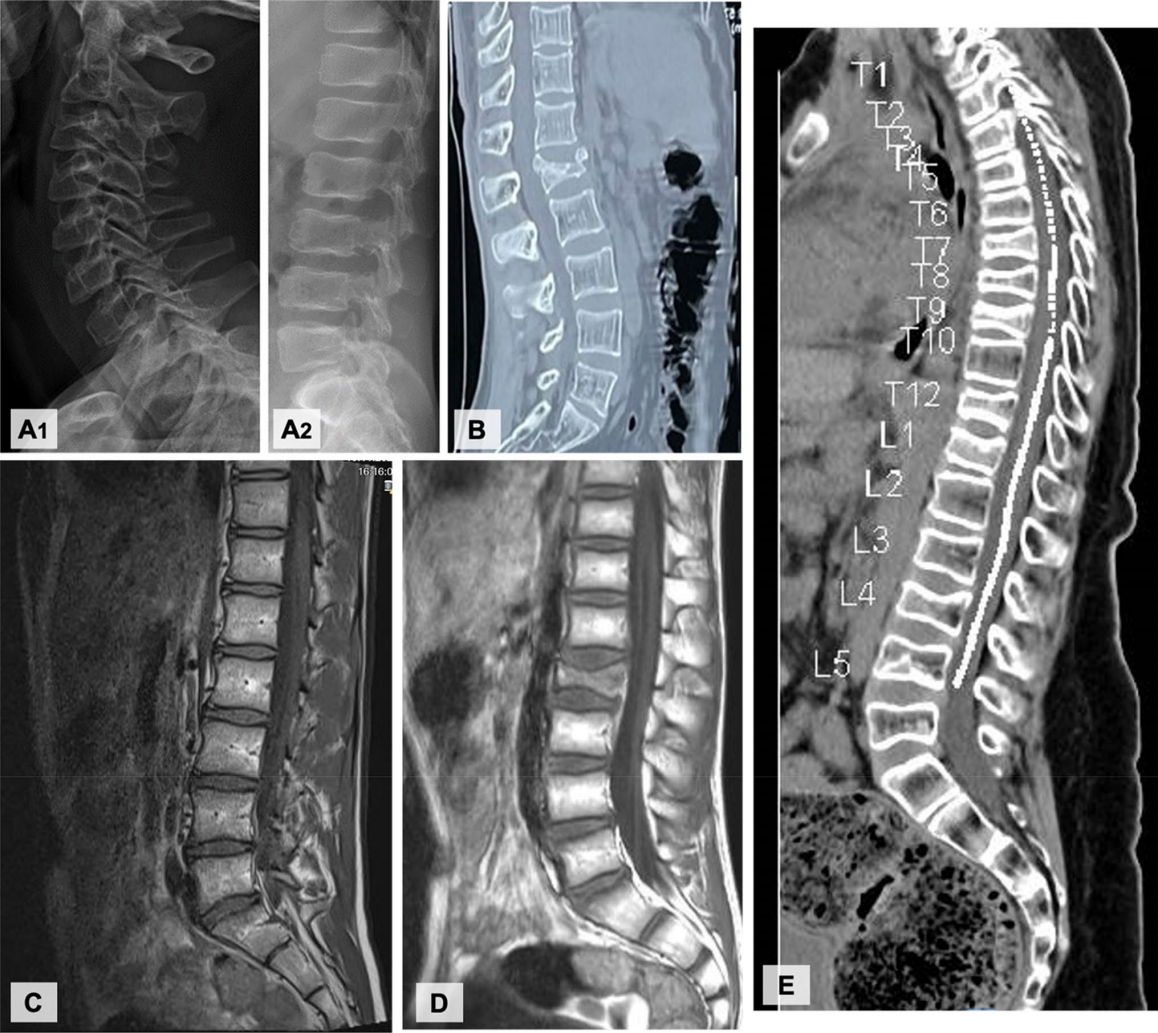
Spinal images: collapse and a reduction of height of multiple vertebral bodies: A Family 1 index patient’s x-ray; B Family 2 index patient’s CT; C Family 3 index patient’s MRI; D Family 4 index patient’s MRI; E Family 5 index patient’s MRI
